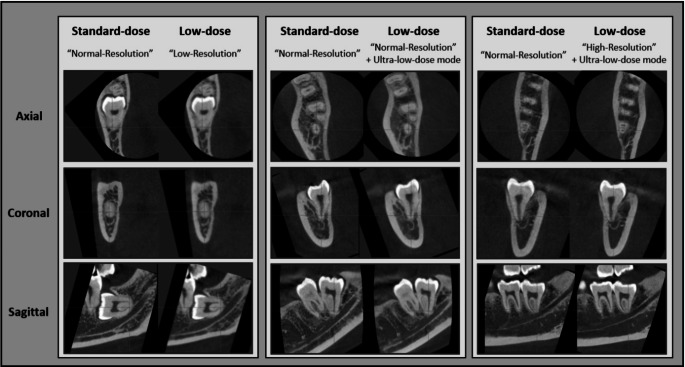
Axial, coronal, and sagittal views of registered paired standard-dose and low-dose CBCT scans. The left, middle, and right pairs display CBCT images acquired using default normal-resolution settings paired with default low-resolution settings, default normal-resolution setting with Ultra-low-dose mode, and default high-resolution setting with Ultra-low-dose mode, respectively
